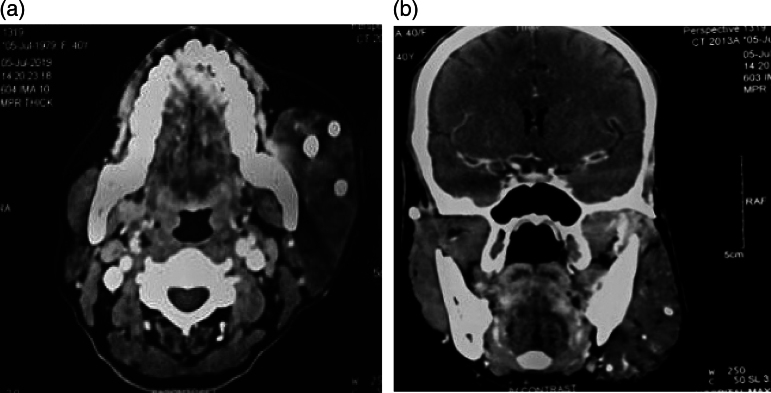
Multidetector computed tomography of the neck with intravenous contrast: (a) axial view and (b) coronal Multiplanar Reconstruction (MPR) view showing combined vascular malformation of size 7.7 × 5.2cm with both venous and arterial components.
